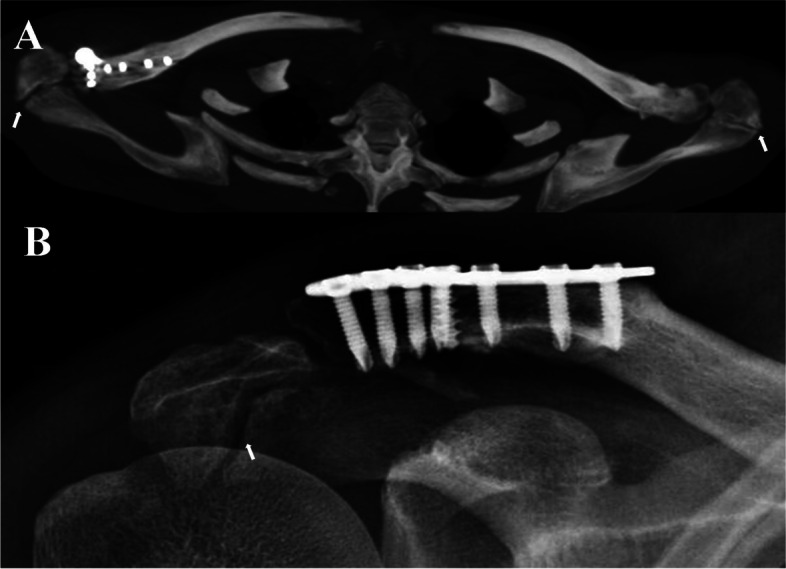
Postoperative CT and X-ray. A Bilateral shoulder CT: the distal clavicle fracture is reduced and fixed using a 3.5 mm distal radius volar plate. The os acromiale is bilateral in this case. B Two years after surgery, the true AP view: the distal clavicle fracture achieved bone union in the anatomic position. The os acromiale can be observed. The white arrowhead indicates the os acromiale
